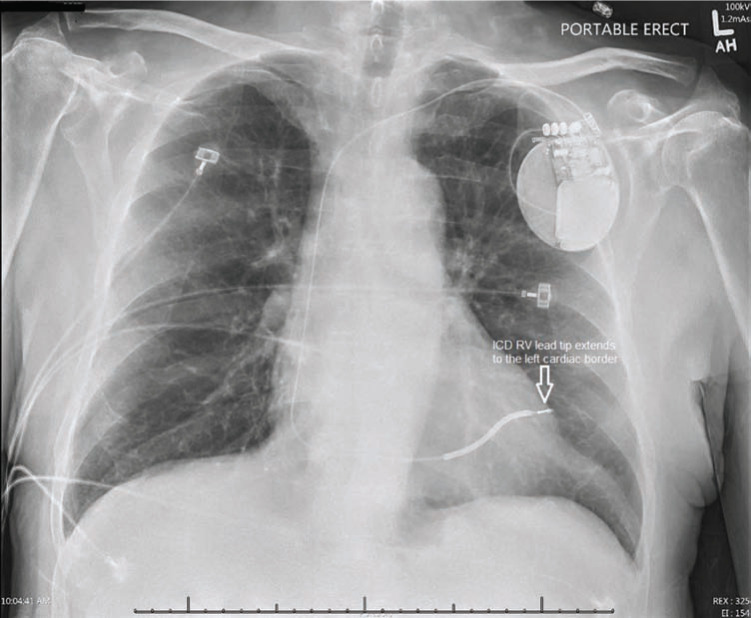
Chest X-ray indicating ICD lead misplacement.
